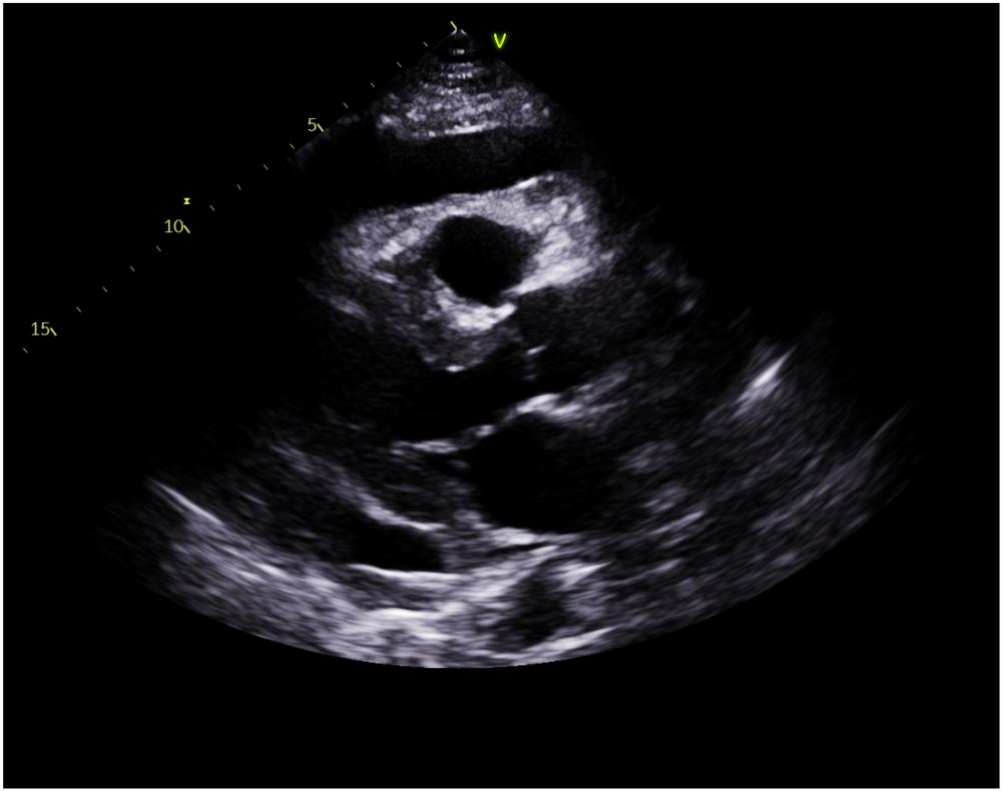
The echocardiogram showed moderate pericardial effusion.
